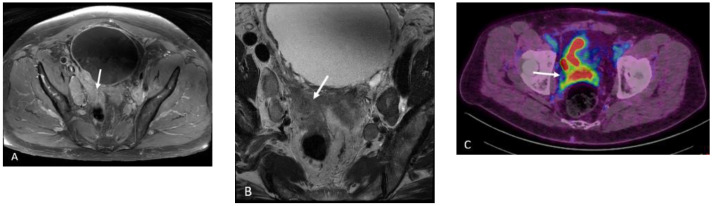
Seventy-nine-year-old male with history of Gleason 9 prostate cancer presenting for initial staging given high risk disease. Axial T1 post-contrast (A) and axial T2 (B) images demonstrate extra-prostatic extension of tumor into the right seminal vesicle and right pelvic sidewall (white arrows). Axial-fused 18F DCFPyL PET/CT (C) demonstrates intermediate- to high-grade activity extending to these regions (white arrows), consistent with miT4 disease.
